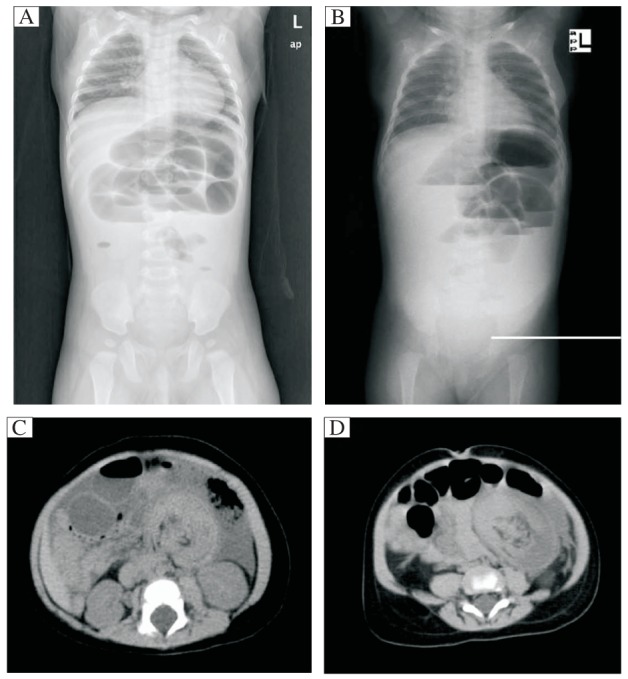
The imaging examination of patients. A and B: Abdominal X-ray showed there was an intestinal obstruction in children. C and D: CT showed that there was an sign of concentric circle with intestinal intussusception preoperatively.
